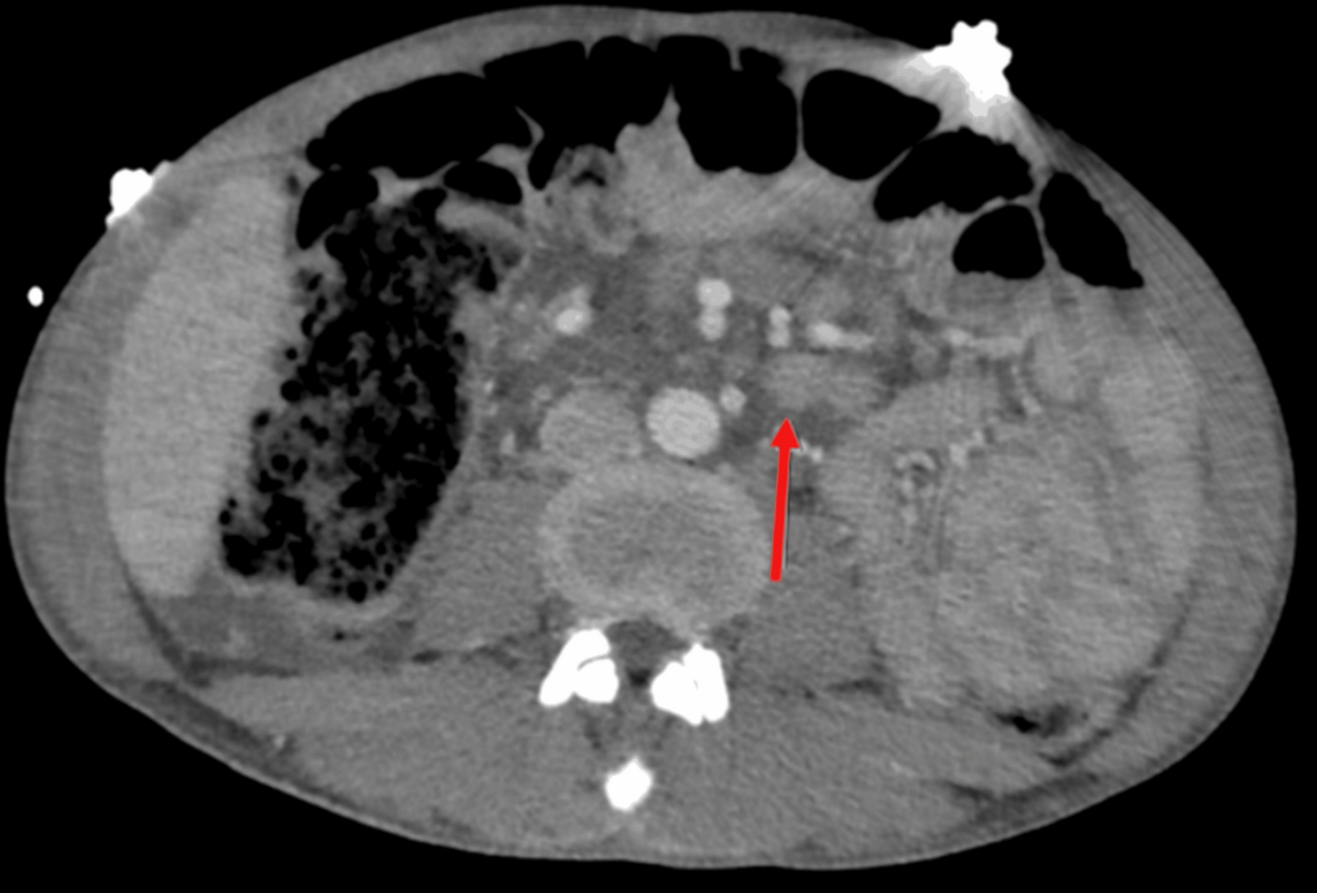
Contrast-enhanced computed tomography scan of the abdomen in the axial plane, showing mesenteric and retroperitoneal lymphadenopathy
